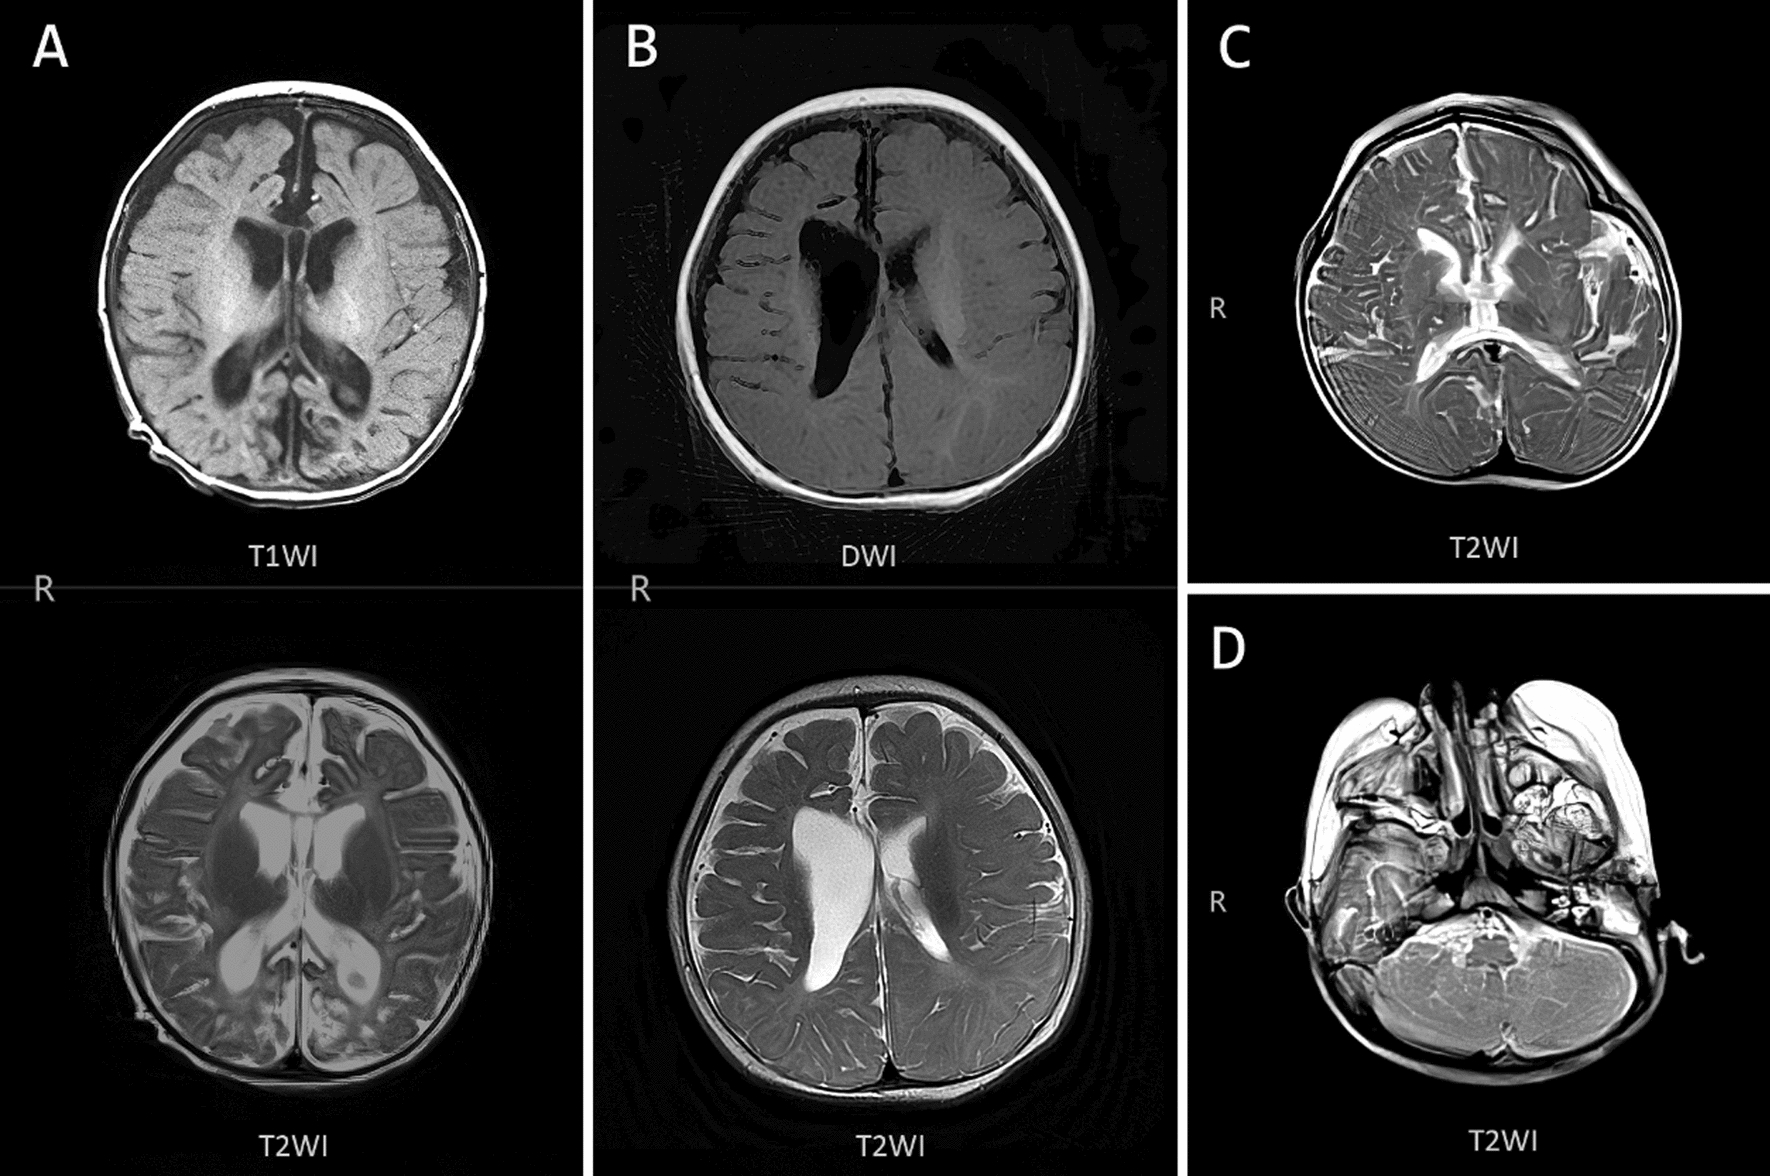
MRI of brain atrophy and encephalomalacia: A The MRI of the 7th patient showed T1- and T2-weighted MRI showing brain atrophy. B The MRI of the 24th patient showed T1- and T2-weighted MRI showing right periventricular leukomalacia. C The MRI of the 41th patient showed T2-weighted MRI showed encephalomalacia at the thalamus. D The MRI of the 41th patient showed T2-weighted MRI showing cerebellar encephalomalacia
